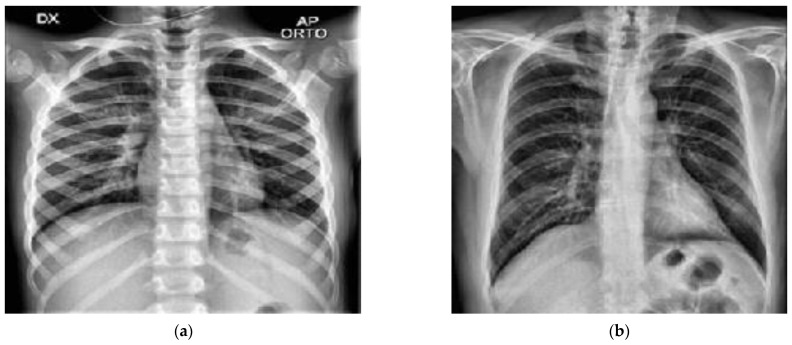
Chest X-ray image data samples. (a) Healthy; (b) COVID-19.
